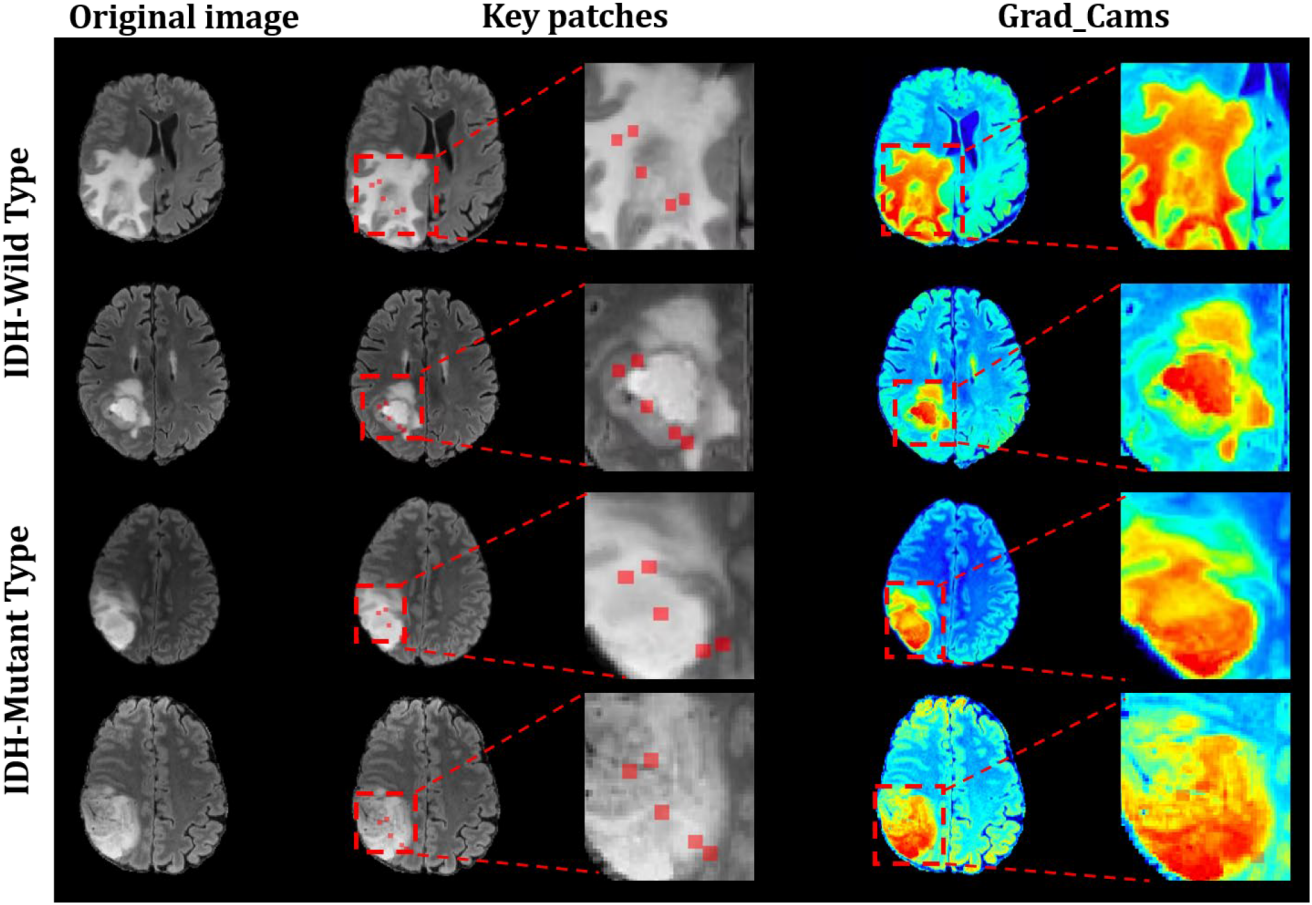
Interpretability of the HAB-MIL model. The first column is FLAIR slices extracted from different patients. Meanwhile, the second column is key patches from HAB-MIL, whereas the third column is their corresponding Grad-CAMs with the HAB-MIL.
